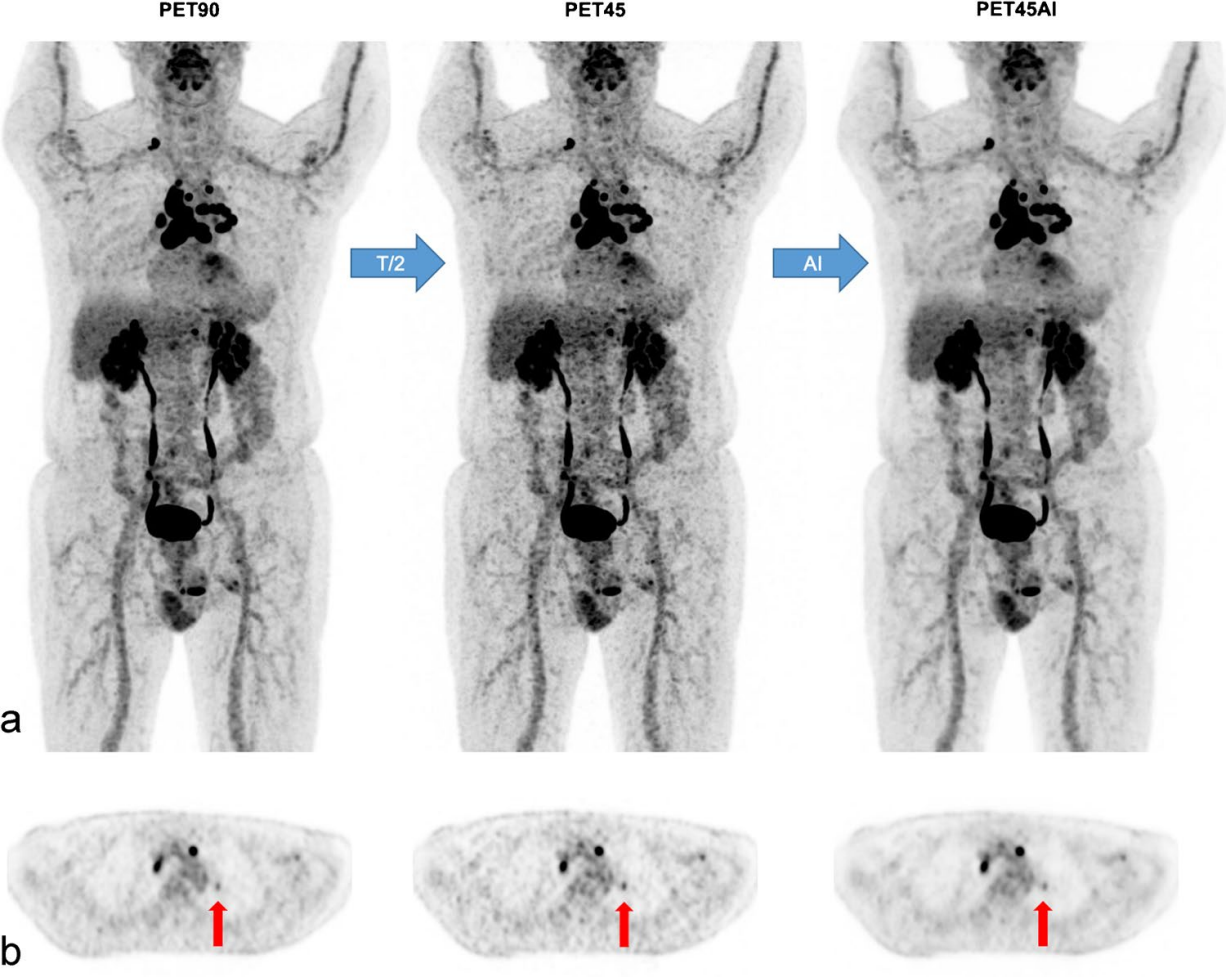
Concordant lesions A 77-year-old man (78 kg; BMI 24 kg/m^2^) with multifocal lymphadenopathy of unknown origin. MIP views (a) and axial PET slices (b) of [^18^F]FDG PET90, PET45, and PET45AI. Detection of small left suprahilar lymphadenopathy in all PET series (vertical arrows in b) with respective standard SUL_max_ of 1.8 (PET90), 2.3 (PET45), and 1.7 g/ml (PET45AI). Nonetheless, PET45 images are noisier than PET90 or PET45AI images, particularly in the liver
